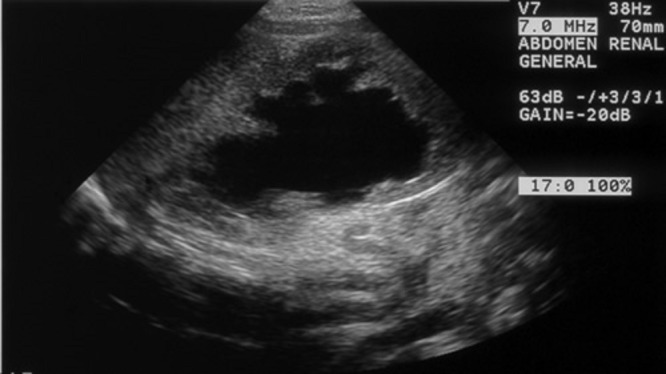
Figure 1: Neonatal renal ultrasound performed prior to operative intervention for prolapse, showing severe left hydronephrosis. Hydronephrosis on the right was comparable in severity.
